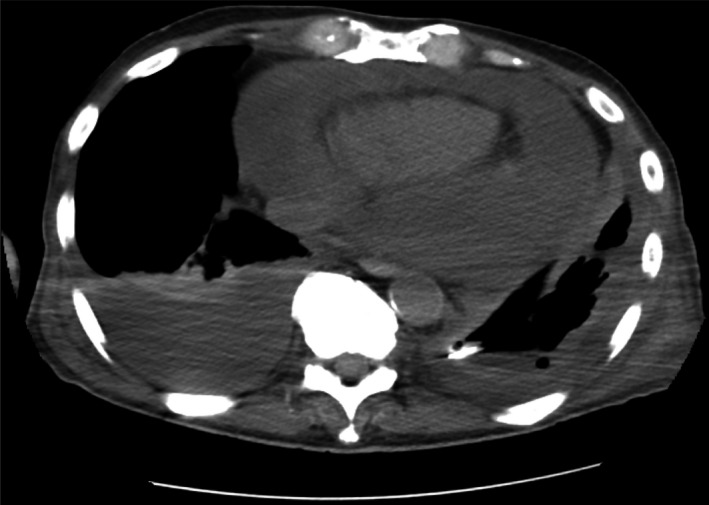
Enhanced chest computed tomography scan obtained at 6 hospital days, showing markedly increased pericardial effusion in the pericardial space, indicating cardiac tamponade
